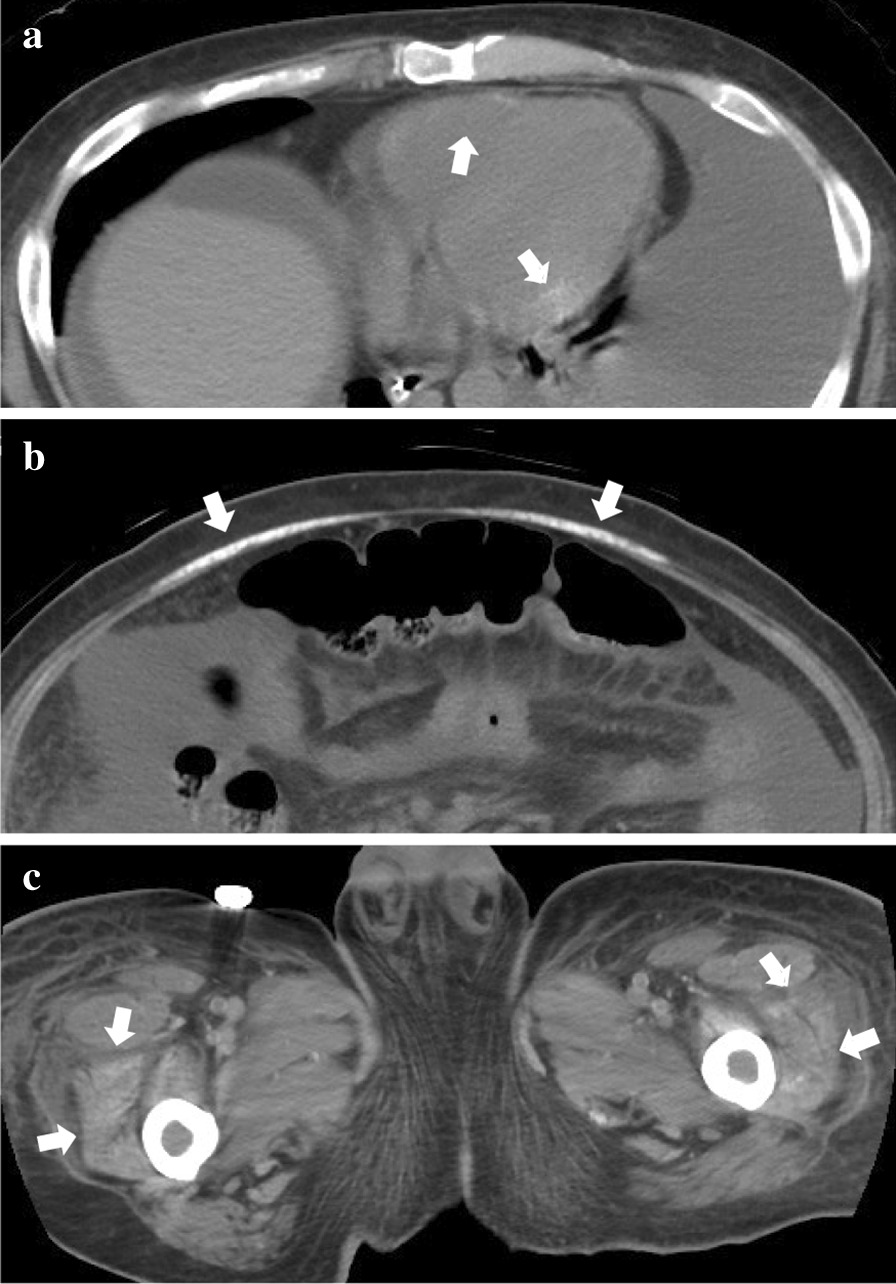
Myocardial and skeletal muscle calcification noted on computed tomography scans for the first time. a High density areas were present on the myocardium (arrows). b, c The rectus abdominis muscle and those of the thigh (arrows) showed high density bilaterally
